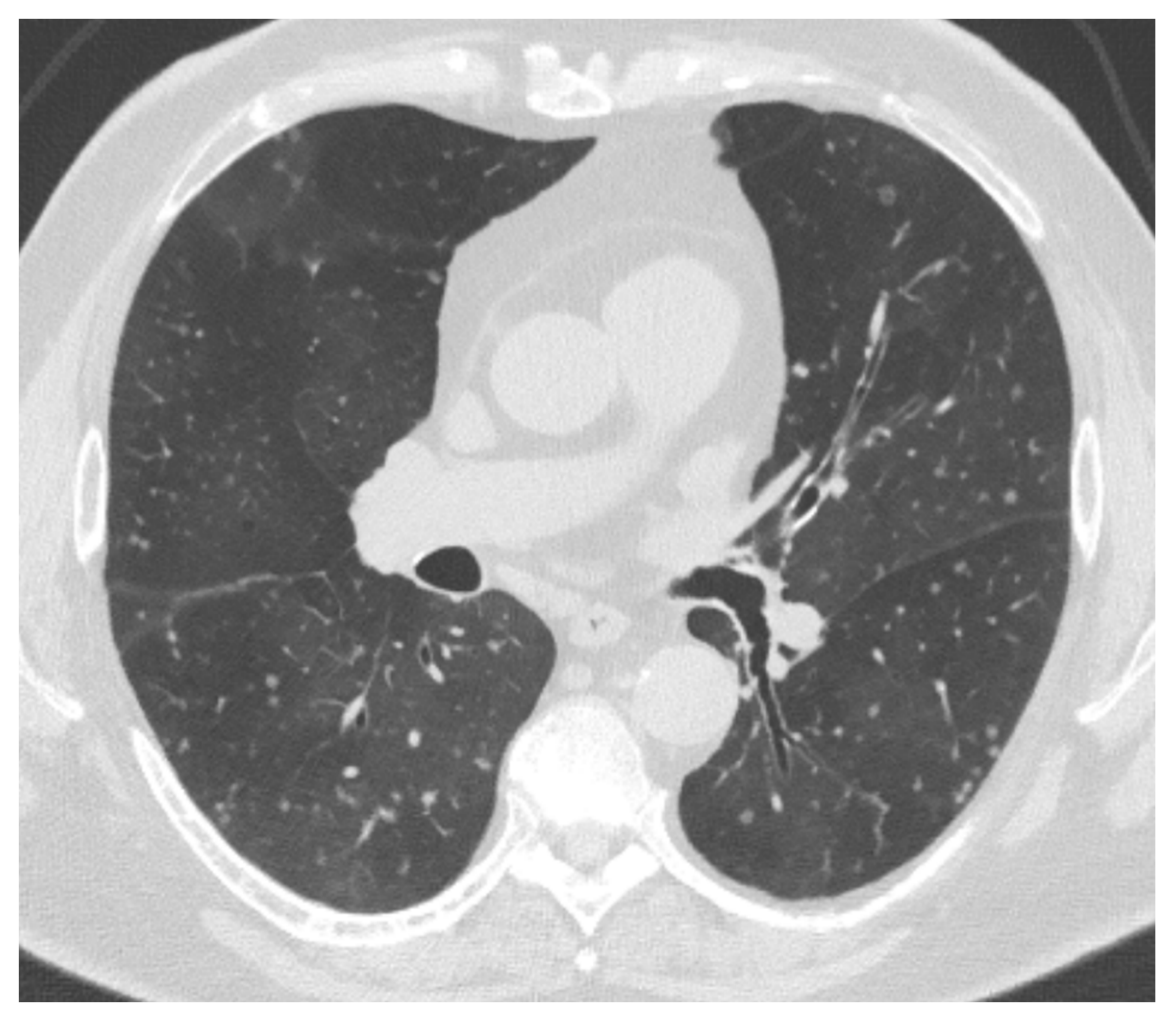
High-resolution chest CT image of a 68-year-old woman, non-smoker, with diffuse idiopathic pulmonary neuroendocrine cell hyperplasia (DIPNECH) syndrome. Multiple pulmonary small nodules are present in the background of mosaic pattern due to patchy air trapping. She had moderate airflow obstruction on pulmonary function testing with a forced expiratory volume in 1 second (FEV
